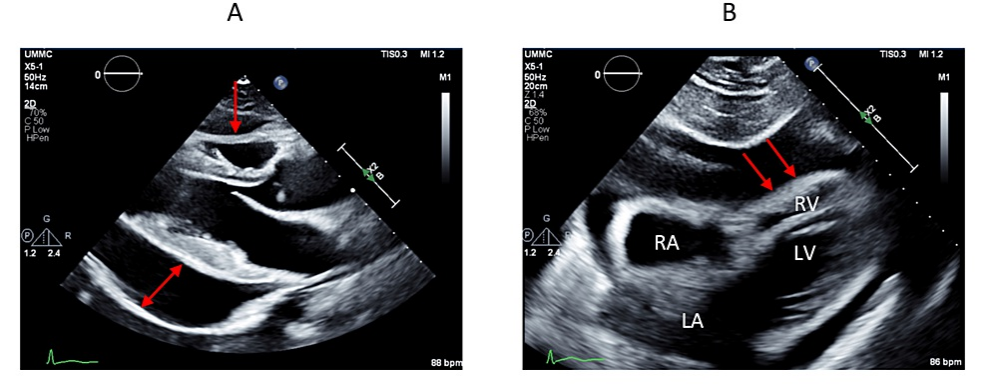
Echocardiography demonstrating tamponade physiology A: demonstrates pericardial effusion posterior to the left ventricle (indicated by the red double arrow) and early diastolic RV collapse (indicated by the red arrow) on the parasternal long-axis view. B: highlights RV collapse during early diastole (indicated by the red arrows) on the subxiphoid view. RA: right atrium; RV: right ventricle; LA: left atrium; LV: left ventricle
